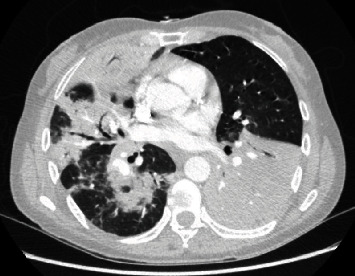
Computed tomography (CT) scan of chest showing consolidation in the left lower lobe, right middle lobe, and right lower lobe with air bronchograms.
